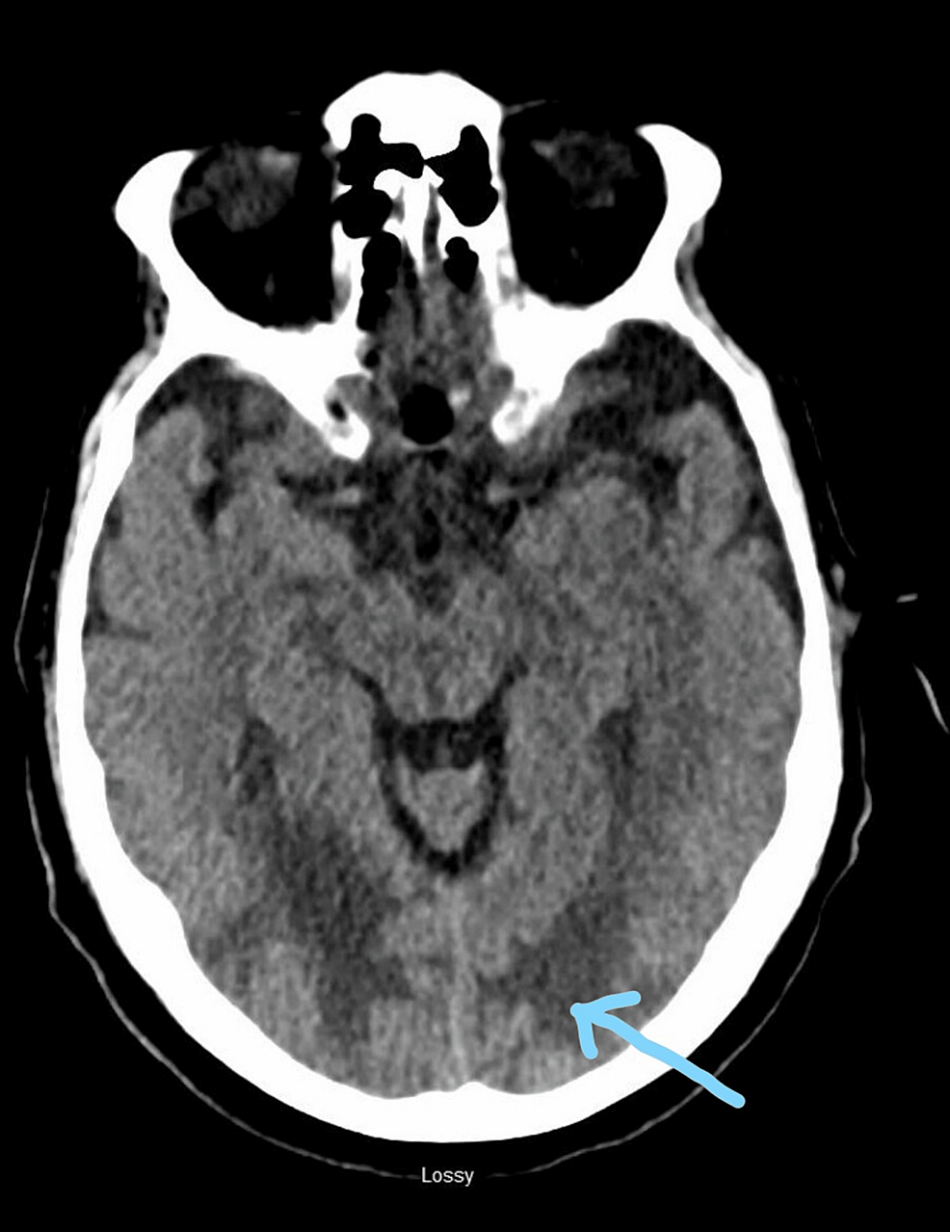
CT head of brain showing extensive hypodensity in bilateral occipital white matter most likely secondary to vasogenic edema (as marked by the arrow)
